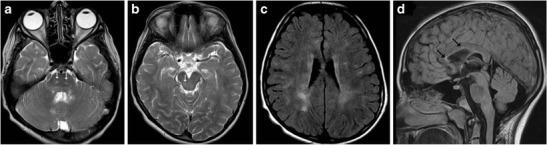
A 14-year-old patient with weakness of the limbs and dysarthria: a–b Axial T2-weighted images show ill-defined increased signal intensity in the pons and midbrain (*arrows*). c Axial FLAIR image shows periventricular white matter hyperintensity. d Sagittal FLAIR image shows increased signal in the callososeptal interface (*black arrows*) consistent with multiple sclerosis
